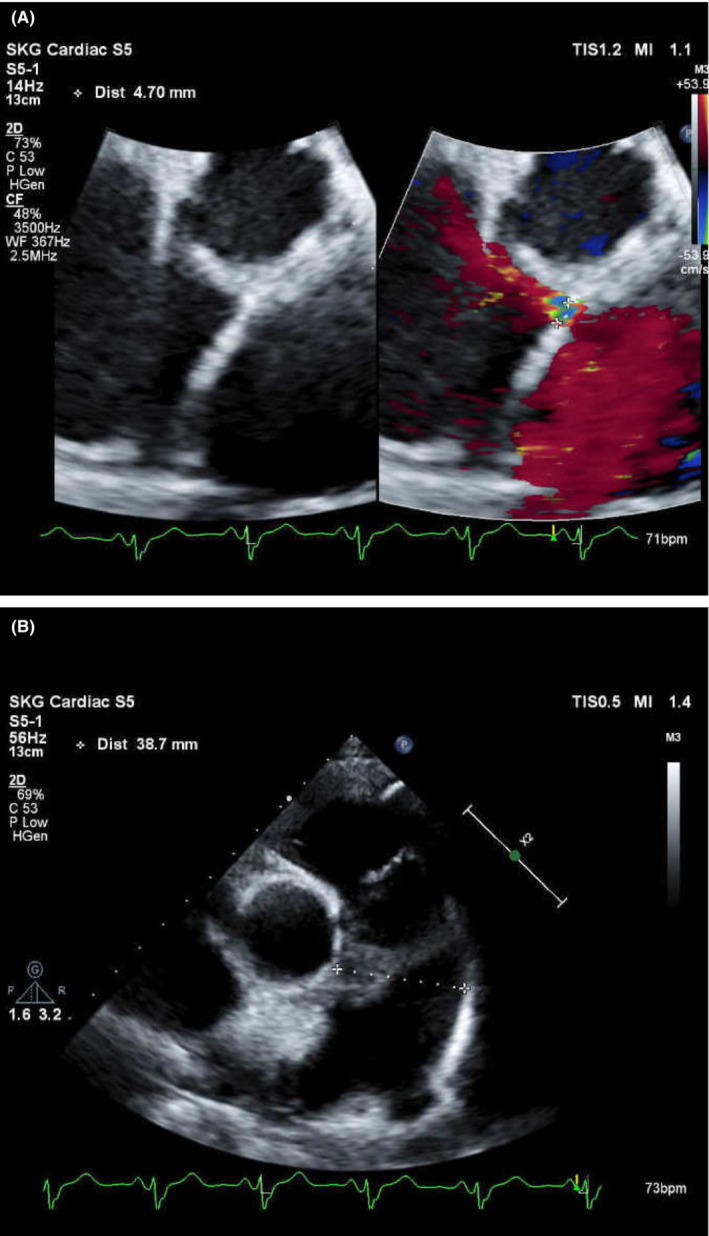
(A, B) Echocardiogram revealed atrial septal defect and pulmonary artery dilation.
